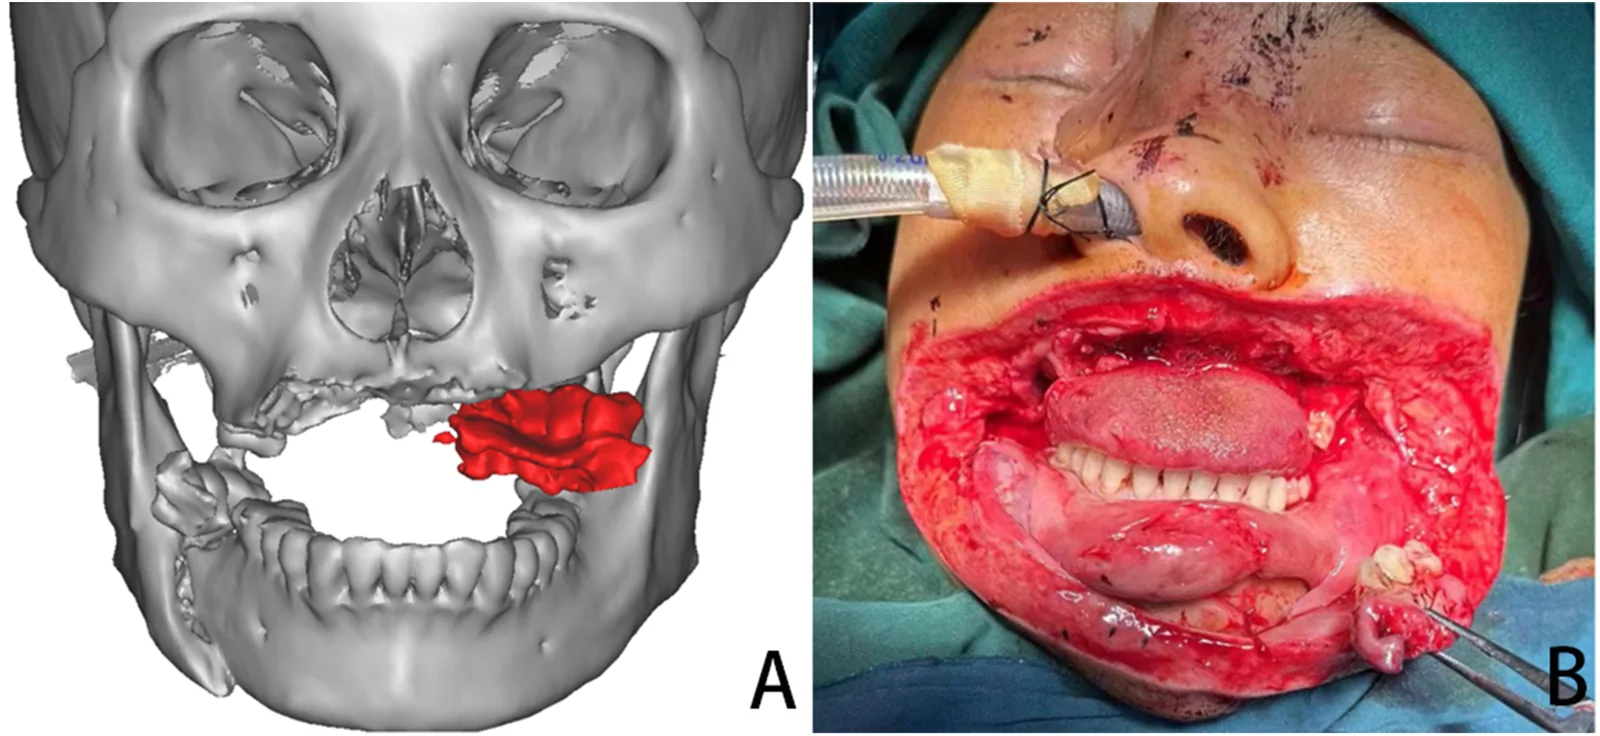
Preoperative and intraoperative images of case 2. (A) Preoperative three-dimensional CT reconstruction. (B) Intraoperative photograph.
